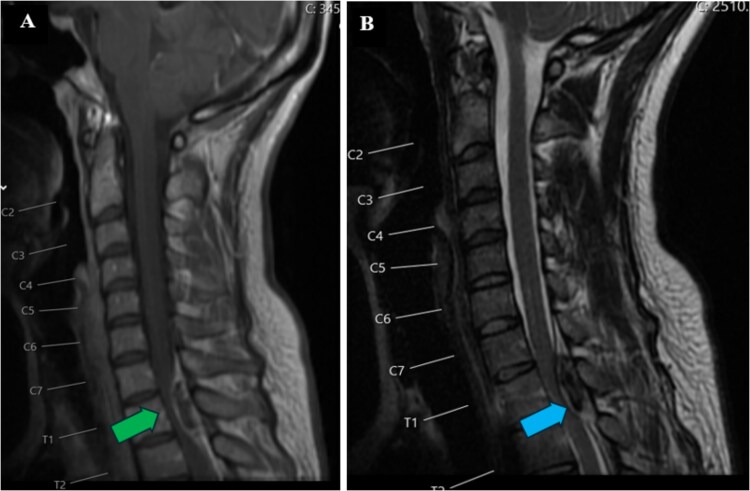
Preoperative MRI of the cervical spine. T1 (A) and T2 (B) sagittal views. Lesion is hyperintense (green arrow) on T1-weighted image and hypointense to isointense (blue arrow) on T2-weighted image at C7-T2 level.
